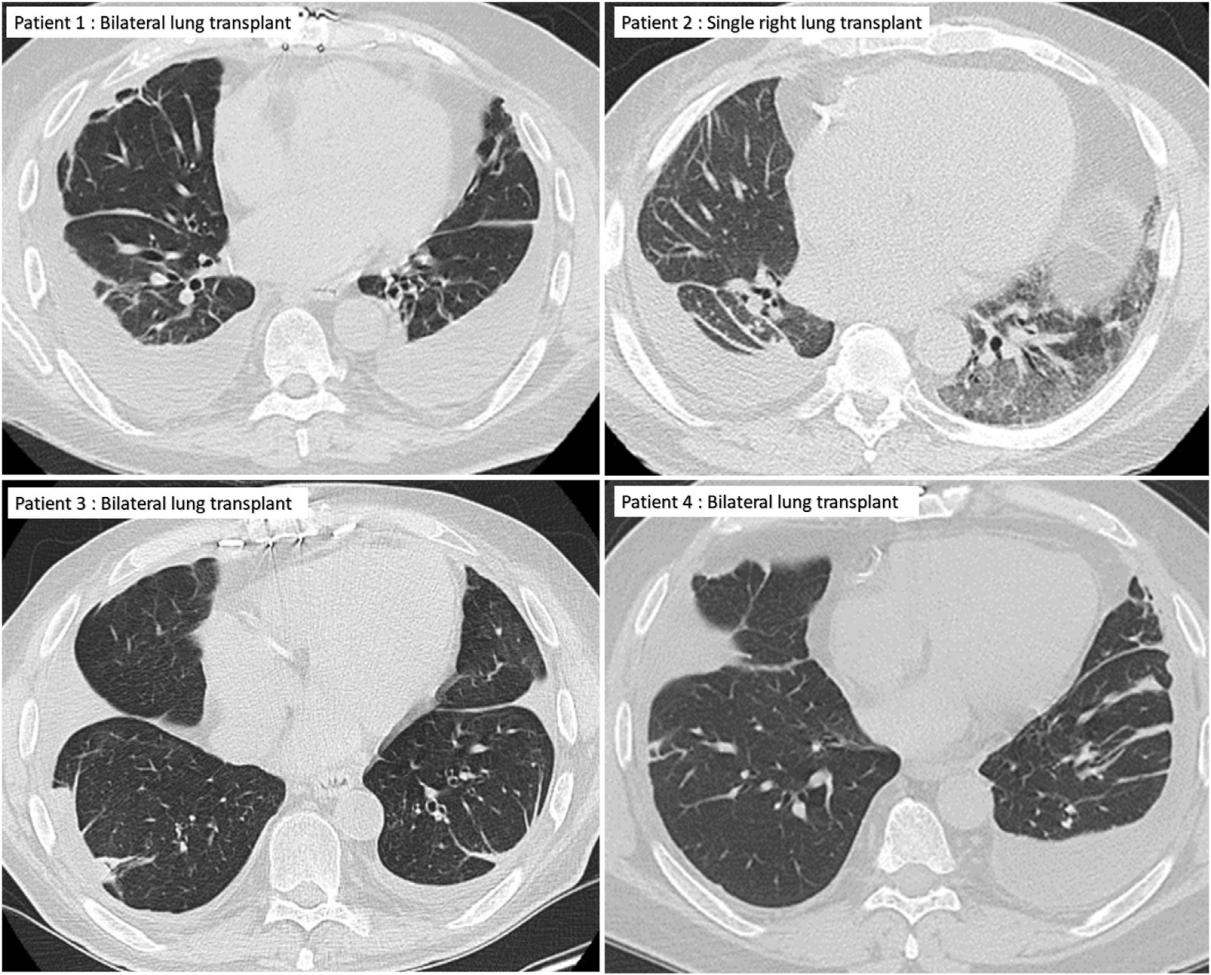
Chest CT of the four patients showing pleural effusions and plate-like atelectasis but no lung parenchymal changes typical of restrictive allograft syndrome.
